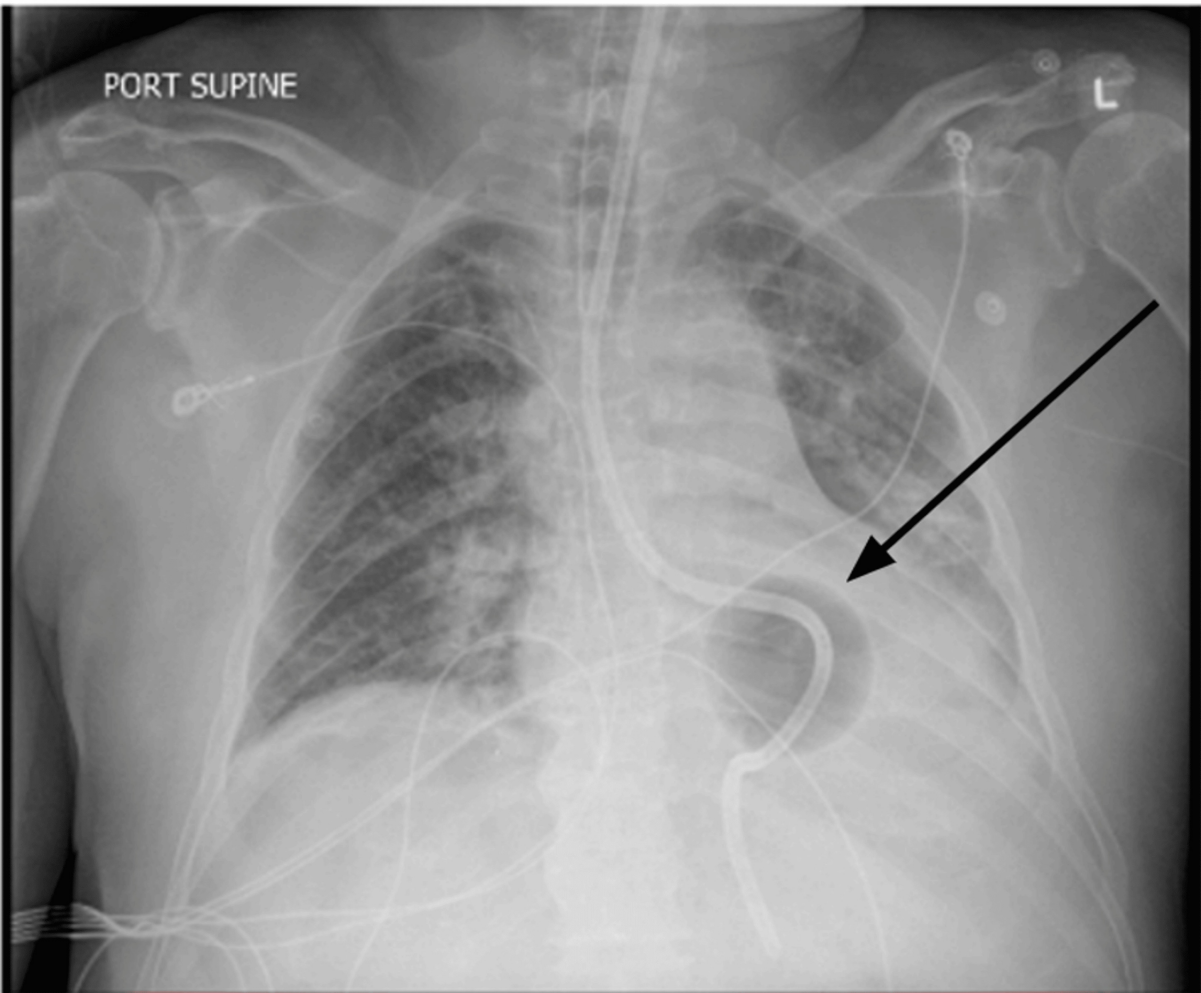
Chest x-ray showing malpositioned Blakemore catheter
